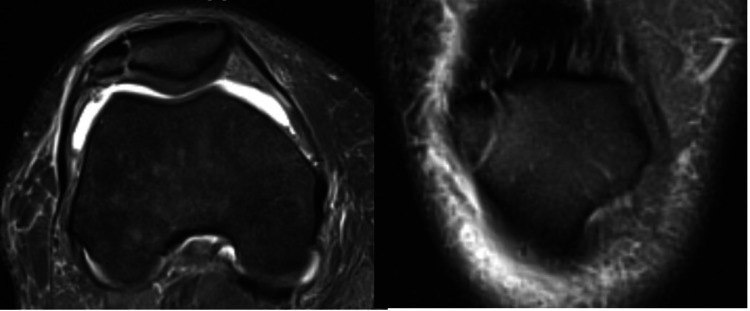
MRI with skyline (left) and AP (right) view of a bipartite patella in the lateral pole (Saupe Type II) in a 47-year-old male presenting with right anterior knee pain and tenderness. Case 6 (Table 1)
